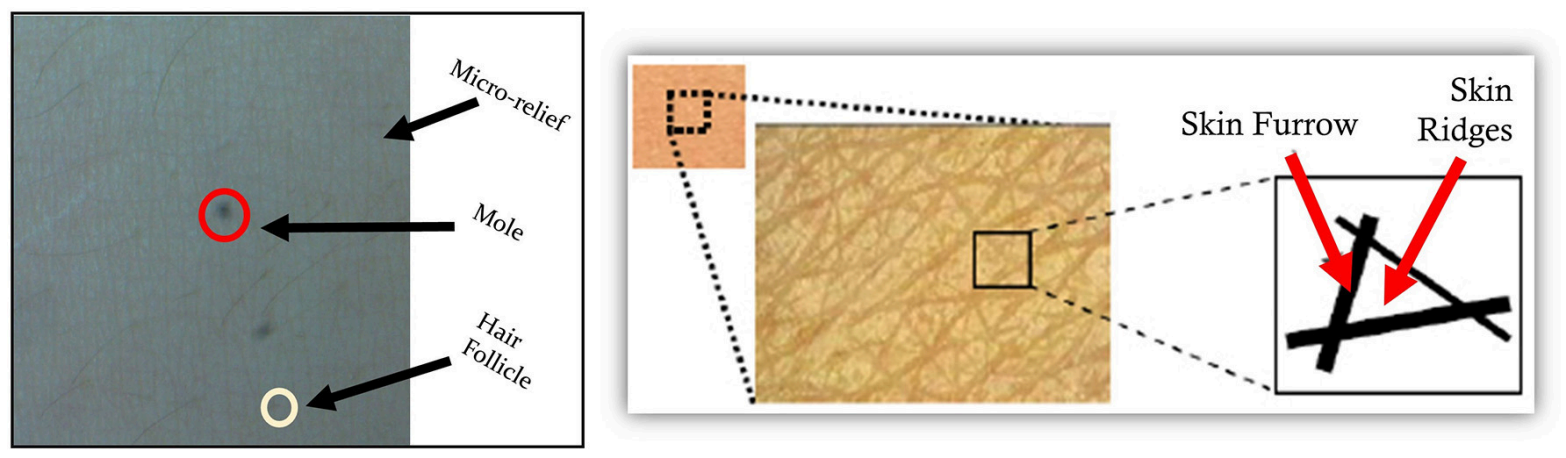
Sound transducer localization using unique skin features. A freehand ultrasound platform featuring an optical camera kinematically coupled to an ultrasound transducer may be used with software that extracts unique skin features and provides transducer localization relative to the skin features. The ultrasound transducer registration facilitates the acquisition of three-dimensional ultrasound volume estimates derived from a standard optically tracked two-dimensional sonograms. This approach allows for scanned images to be generated and reformatted in any plane to allow for the comparison of matched images across serial examinations.
